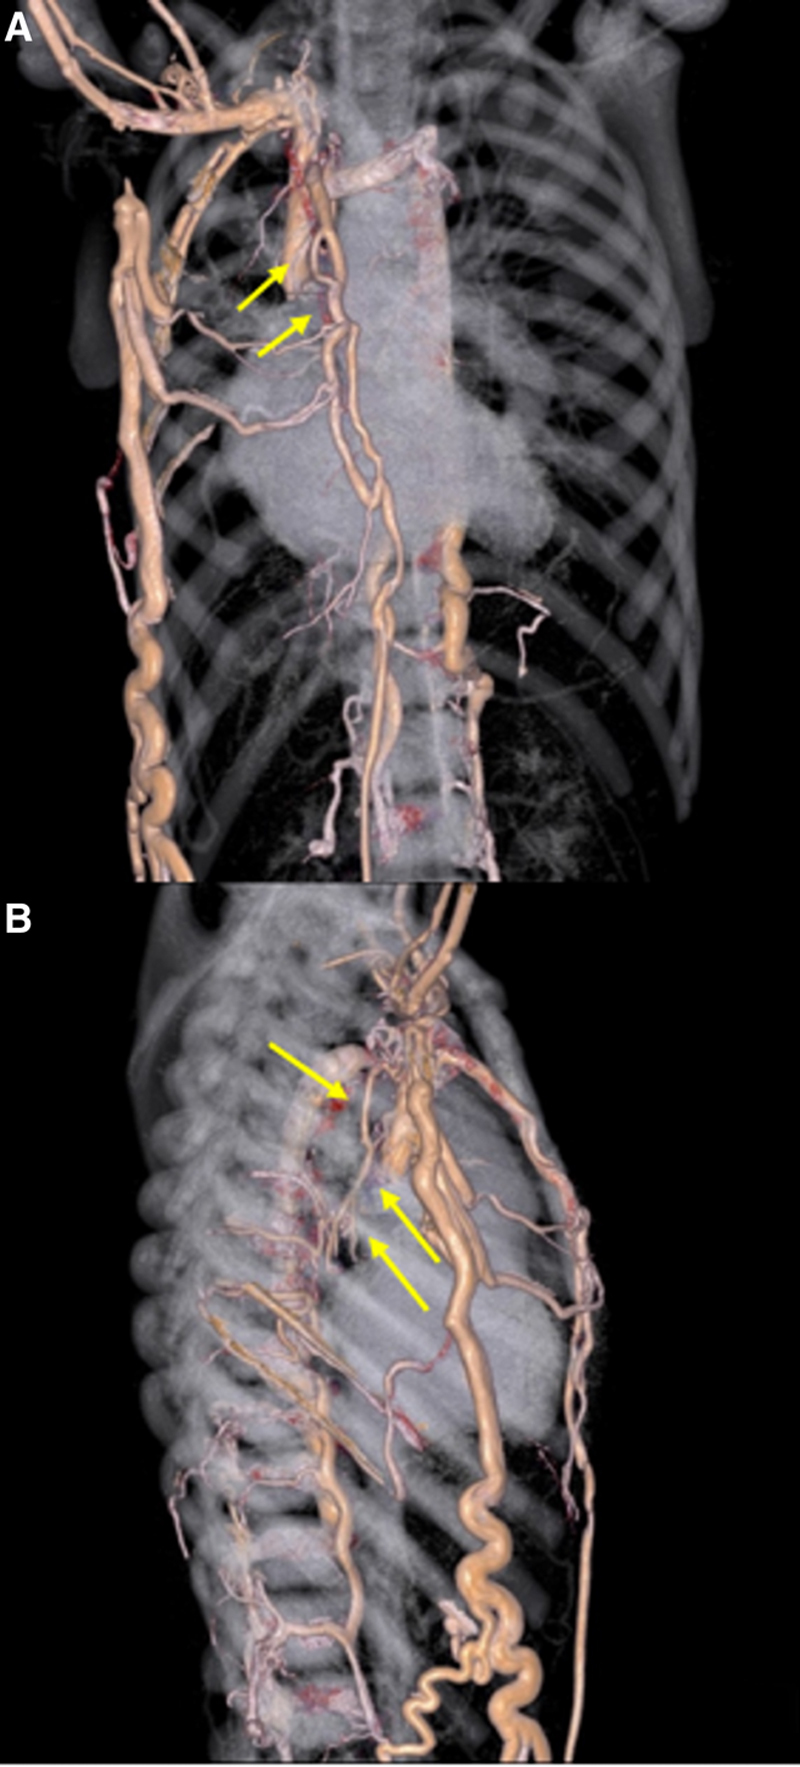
Extensive collateral network on CTA in patient 1. A, Three-dimensional computer tomography angiogram of the thorax from patient 1 showing extensive venovenous collaterals in the paraesophageal region (arrows). B, Lateral 3D image showing network of collaterals (arrows) surrounding mainstem bronchi and with tributaries to intercostals, anterior chest wall and azygous.
